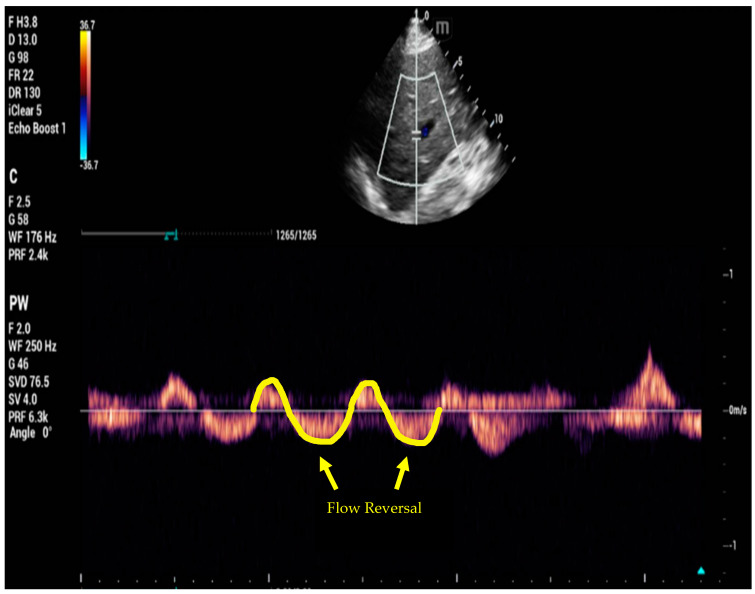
Doppler of hepatic vein showing flow reversal.
